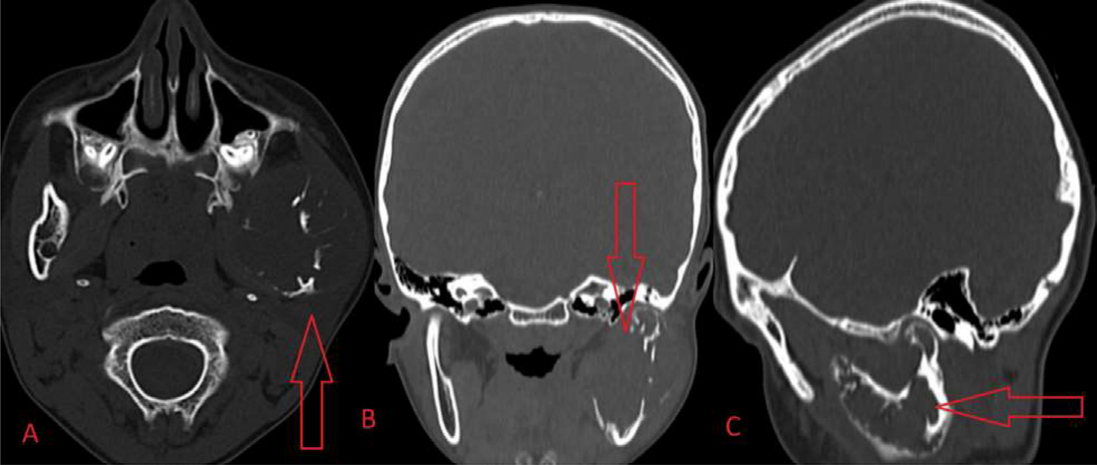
CT bone window in axial (A), coronal (B), sagittal (C) sections showing expansile osseous cystic lesion causing erosion and periosteal elevation measuring 4.6 × 4.5 × 5 cm noted involving left angle, ramus, condylar and coronoid process of left hemimandible.
